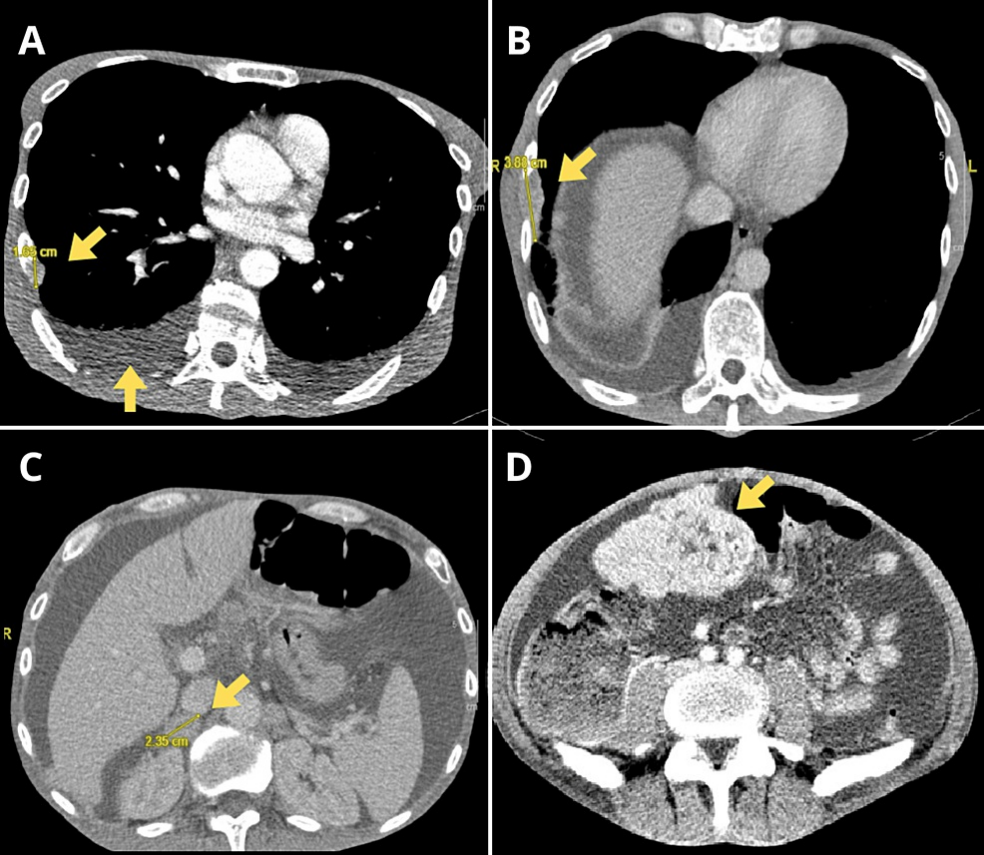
Thoracoabdominal CT, December 2020. Tumor progression, pleural effusion (A, B), ascites (C), and peritoneal carcinomatosis (D) are observed.
